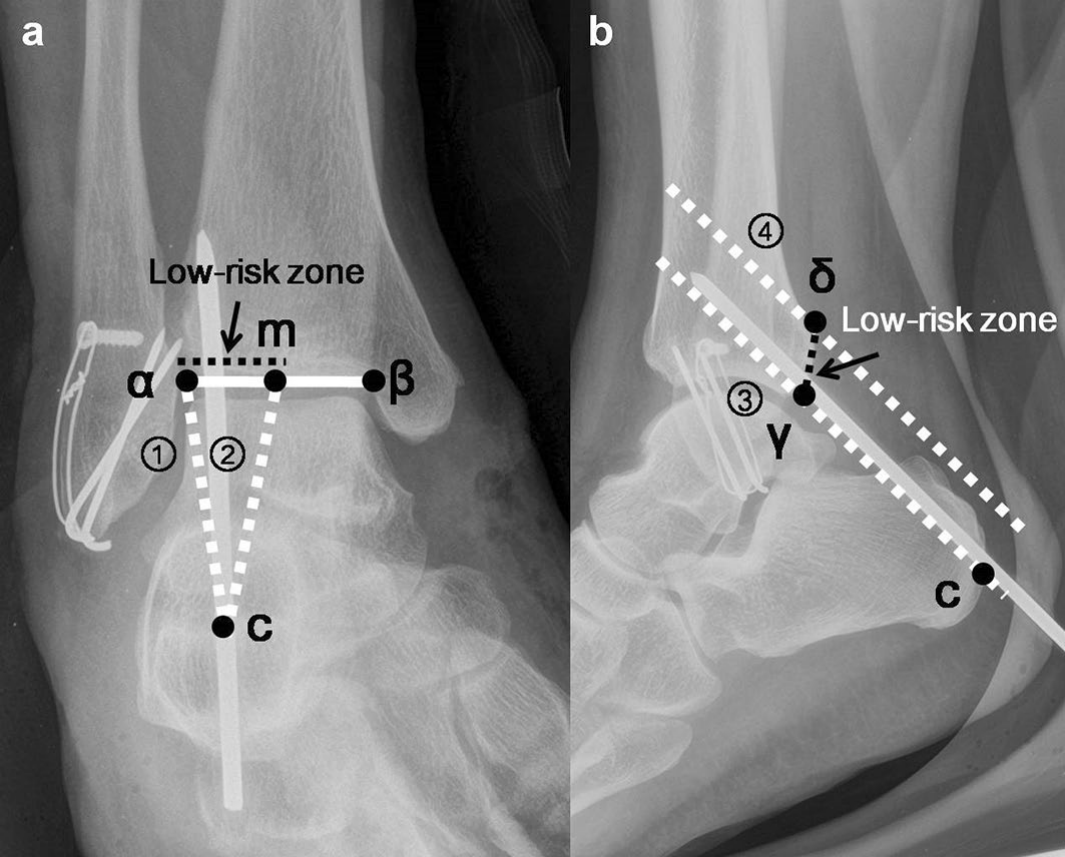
(a) We assumed that it will be safe to insert a pin from the center of the calcaneal tuberosity (c) to the distal tip of the posterior malleolus through the posterior extra-articular ankle space when it is inserted medial to the lateral end of the posterior malleolus (α) (lateral border of the low-risk zone:①) and lateral to the midpoint of the anterior distal tibial articular surface (m) (medial border of the low-risk zone:②) at the joint level on the C-arm fluoroscopic ankle mortise radiograph. (b) On the C-arm fluoroscopic lateral ankle radiograph, pin insertion from the center of the calcaneal tuberosity (c) to the distal tip of the posterior malleolus (γ) corresponded to the inferior border of the low-risk zone (③) and the line parallel to the inferior border of the low-risk zone and 1 cm proximal (δ) was the superior border of the low-risk zone (④). The arrows indicate the low-risk zone.
